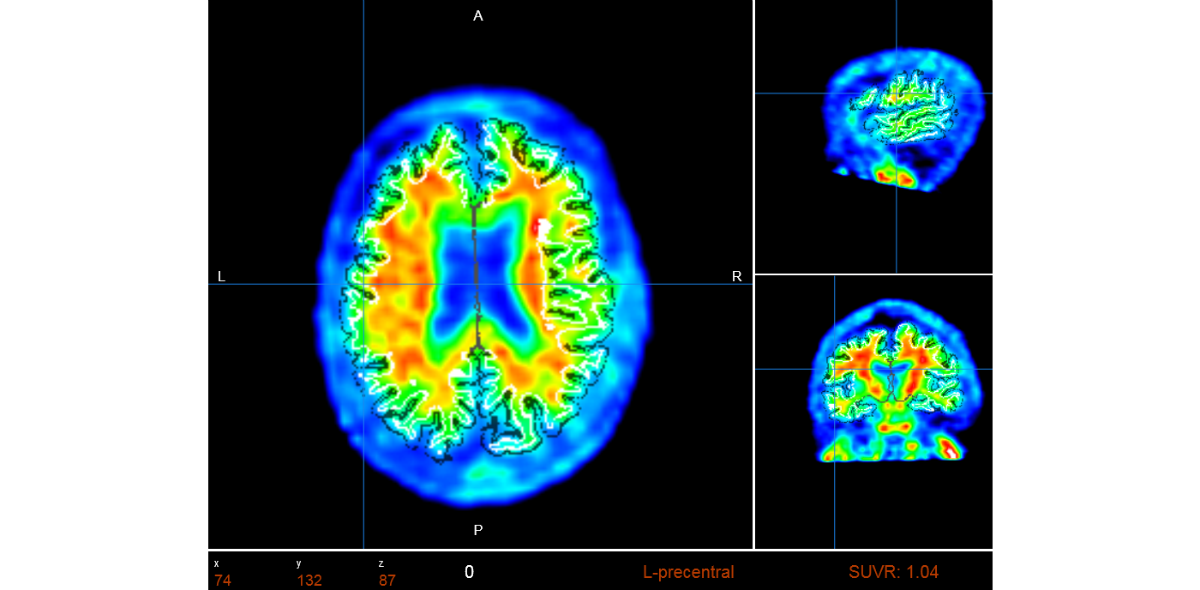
Positron emission tomography (PET) image is overlaid with the white matter surface (shown in White) and gray matter surface (shown in Black). Selected region’s standardized uptake value ratio (SUVR) and name of region are displayed in Red at the bottom of the screen.
